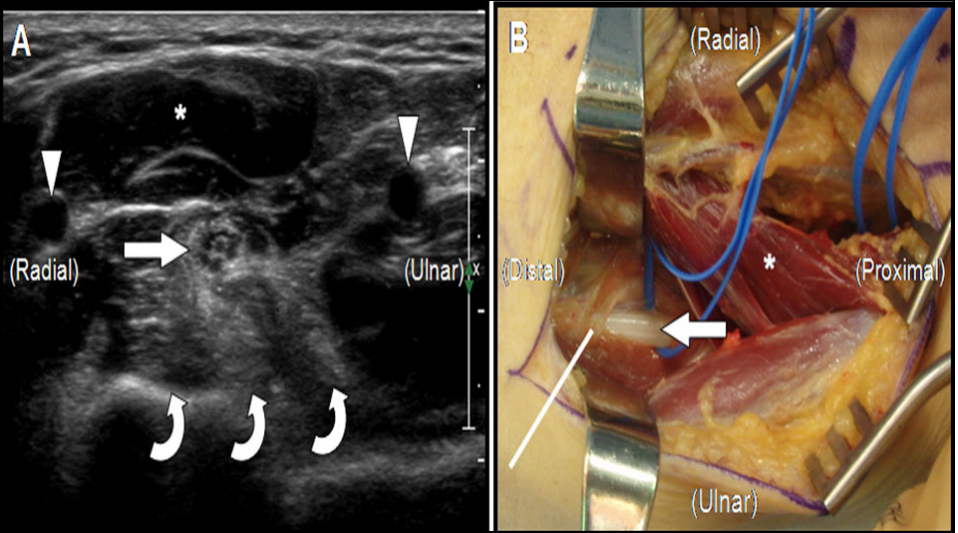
The cross-sectional ultrasound image (A) of the proximal forearm demonstrates the normal echo-texture of the median nerve (arrow). The hyperechoic and homogenous ground glass appearance of the flexor digitorum profundus muscle (curved arrows) is also shown. The intra-operative photo (B) depicts a fibrotic band (straight line) across the anterior aspect of the median nerve (arrow). Arrowheads = arteries, * = pronator teres muscle. The ultrasound image was obtained with a Philips iU22 scanner (Philips Medical Systems, Bothell, WA) with a 12 MHz linear array transducer.
